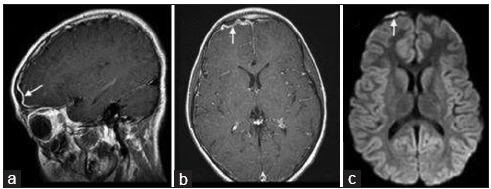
A 9-year-old boy with frontal sinusitis and pneumocephalus found to have an epidural abscess. (a) Sagittal and (b) axial gadolinium-enhanced T1-weighted MR images of the brain demonstrate a contrast-enhancing fluid collection (arrow) overlying the right frontal lobe. (c) The diffusion-weighted image demonstrates restricted diffusion, compatible with an epidural abscess (arrow).
